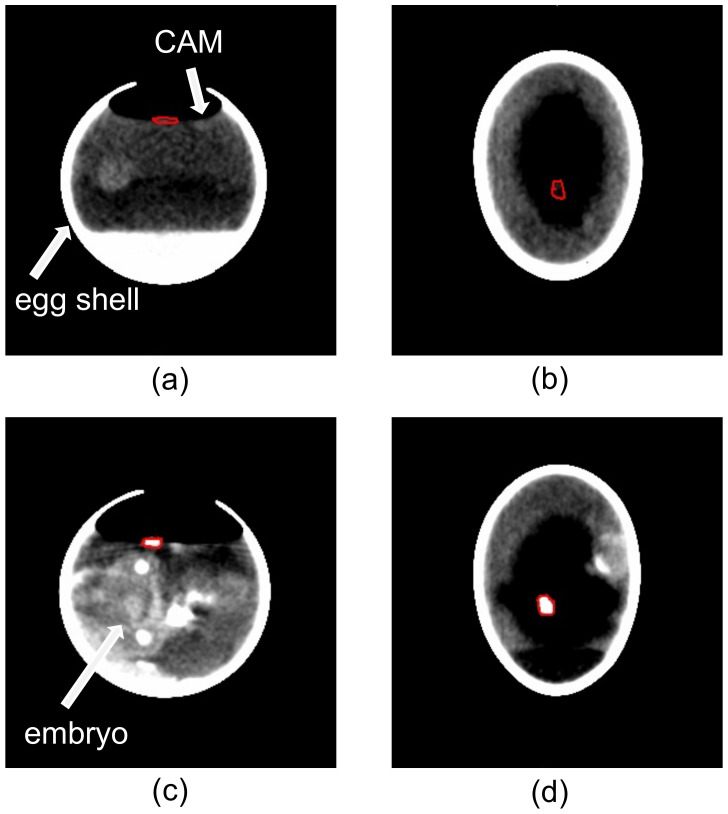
CT-based volume measurement of a cystic tissue depicted in the cyst window (*n* = 36). The cystic tissue is marked by the red border in each image. The chicken egg with engrafted cystic tissue is shown one day after engraftment in the transverse (a) and coronal (b) planes without any signs of calcifications. A significant increase in hyperdense structures in the cystic tissue was visible in the transverse (c) and coronal (d) planes of the second measurement, which took place hours before removal of the cystic tissue.
